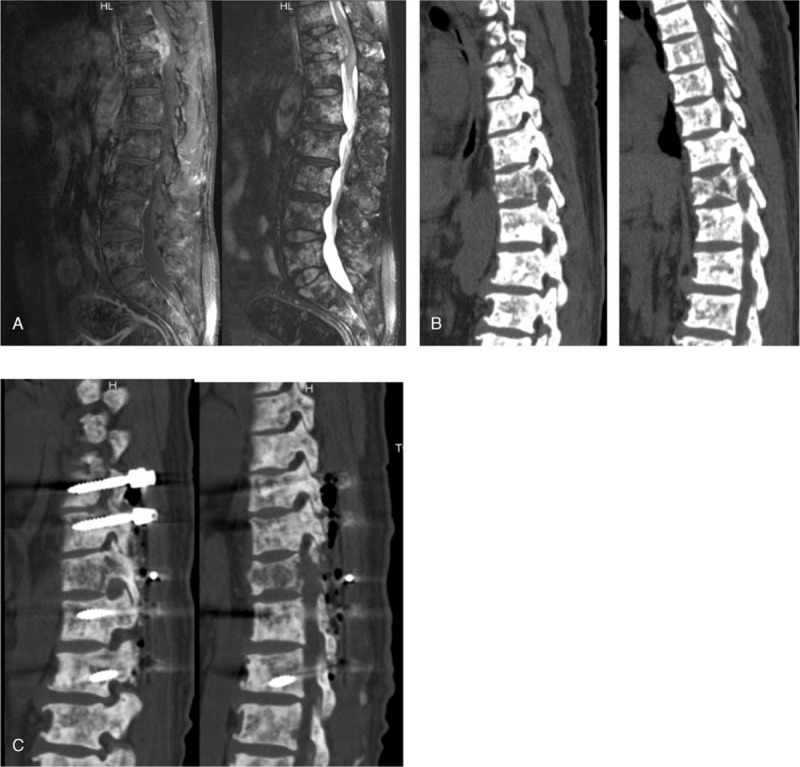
Imaging changes before and after T11 vertebral fracture leading to spinal cord compression and incomplete paralysis. (A) MRI findings showed that the lumbar vertebra was flattened and wedged to different degrees, the T2WI signals of the vertebra and the attachment were uneven and presented diffuse mixed signals of high and low levels, and the fatty inhibition T1WI enhanced scan sequence showed obvious uneven enhancement of the thoracic vertebra 11 and 12. Three-dimensional CT reconstruction, before surgery (B), 10 days after surgery (C).
